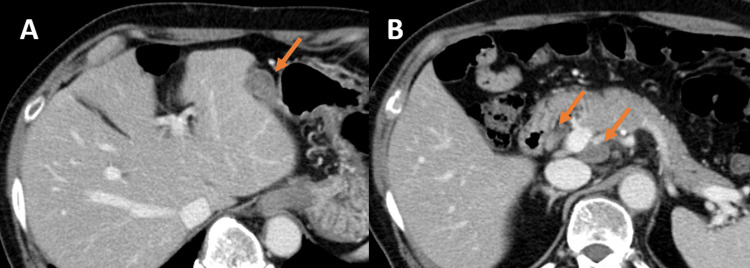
Abdominal contrast-enhanced CT after treatment The CT after five months of treatment showed that (A) the mass between the liver and stomach had shrunk to <2 cm, and (B) the intra-abdominal lymph nodes had also shrunk.
